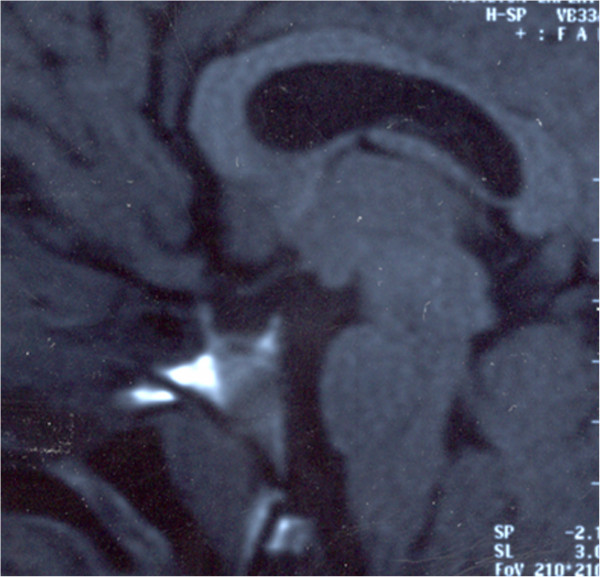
Sagital T1W magnetic resonance image shows a small optic chiasm, a hypoplastic pituitary stalk, an “empty” sella and a partial hypoplasia of corpus callosum.
